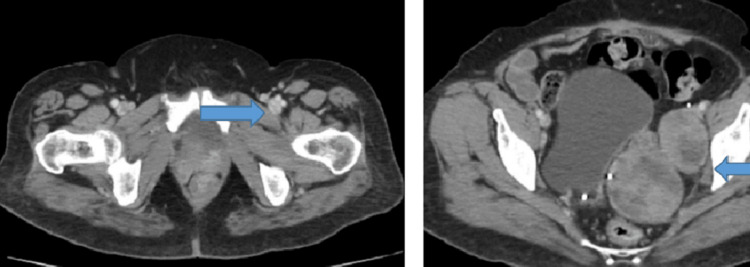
CT scan axial views showing an interval increase in the size of complex lobulated predominantly solid left adnexal mass inseparable from the pelvic side wall and right external iliac lymphadenopathy.
